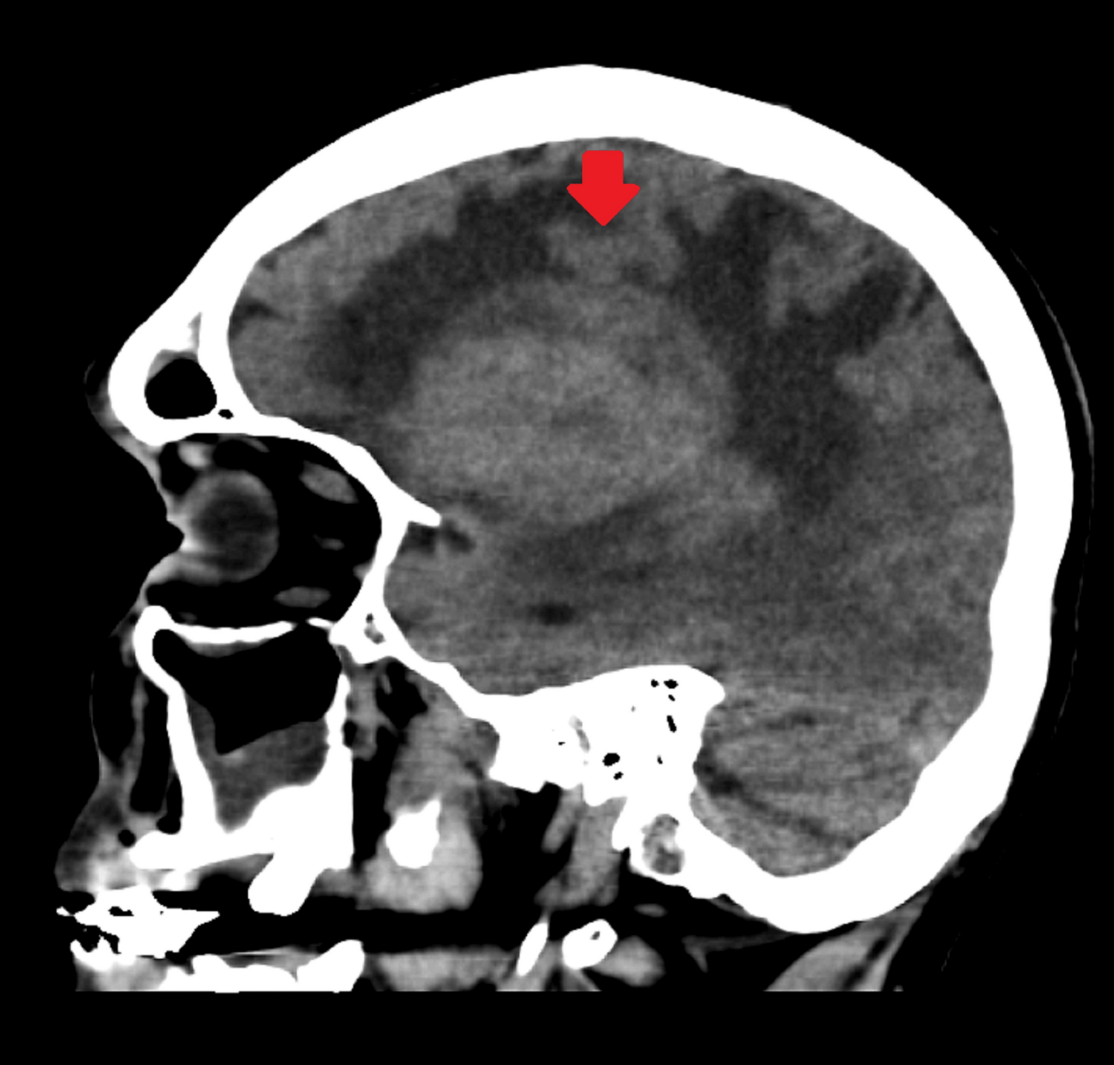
Sagittal section of CT of the brain shows a brain mass
